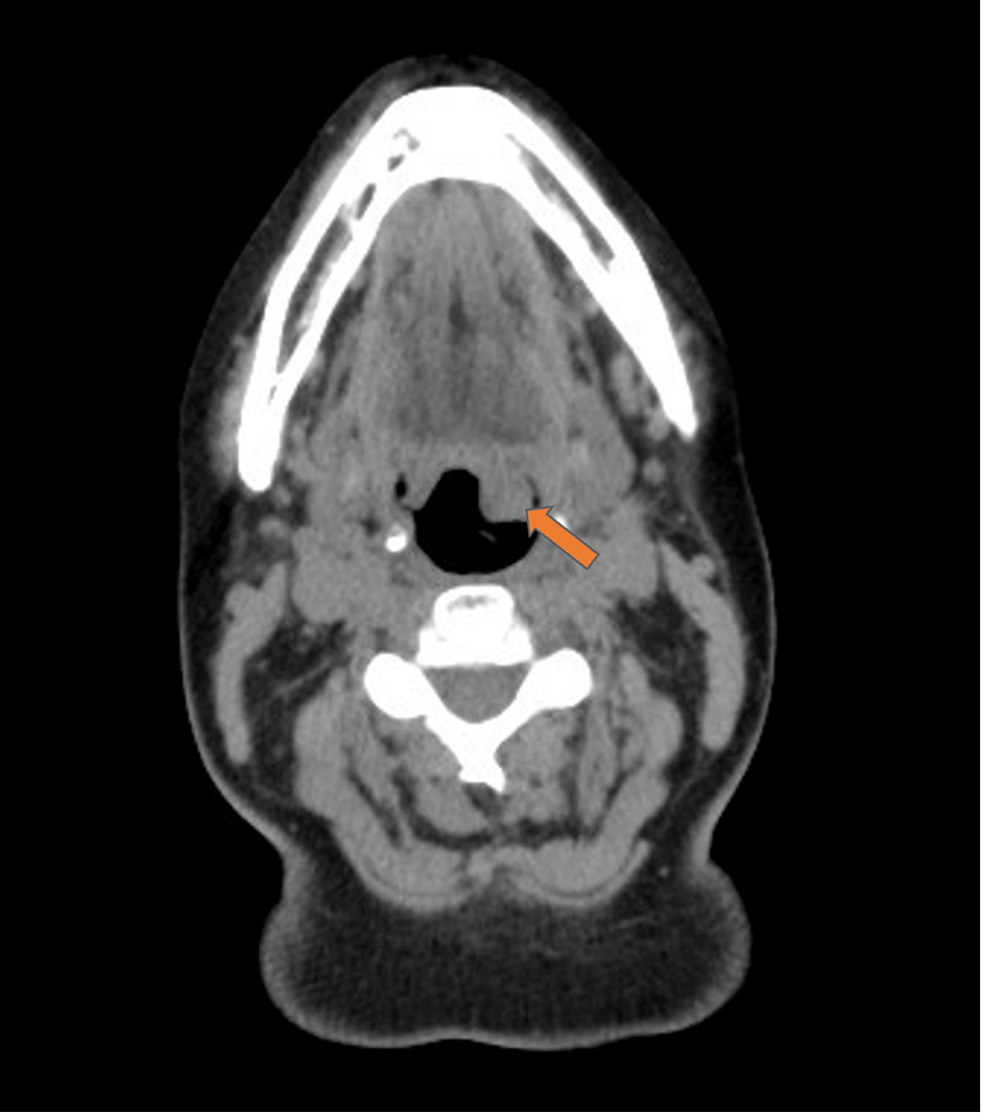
Computed tomography showing the base of the tongue nodular lesion (arrow).
